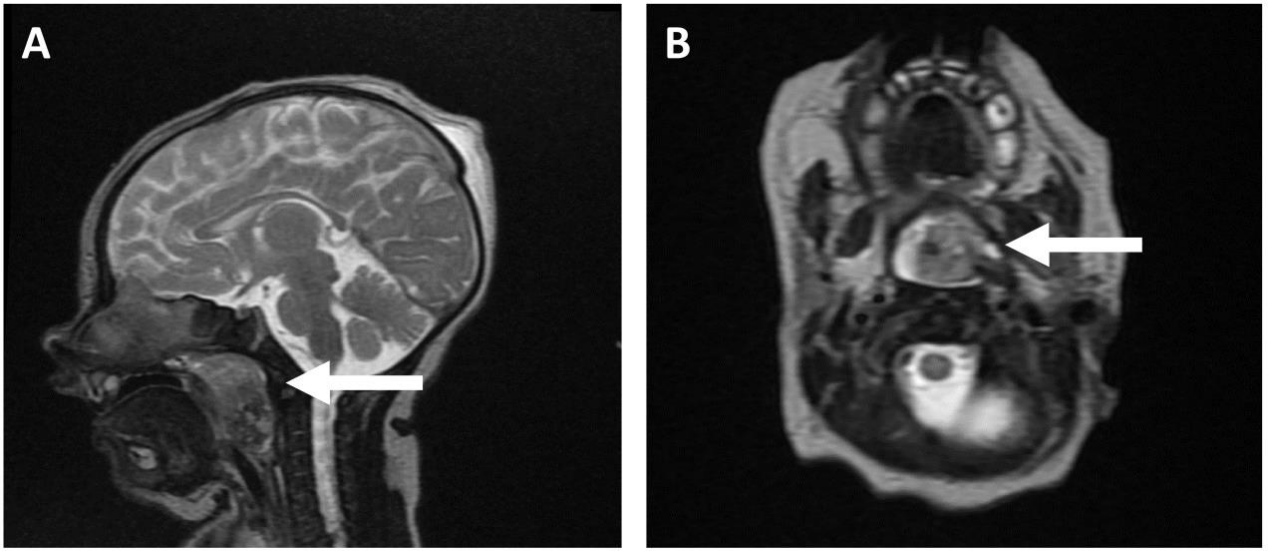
MRI with a Nasopharyngeal Mass Bulging into the Oropharynx and the Base of Tongue, with No Intra-cranial Involvement. A: The lesion consists of restricted areas, areas with decreased susceptibility-weighted imaging (SWI) values. B: Posteriorly, there is a 0.5 cm area with a high T1-weighted signal, and a medium-to-low signal on fat-suppression.
